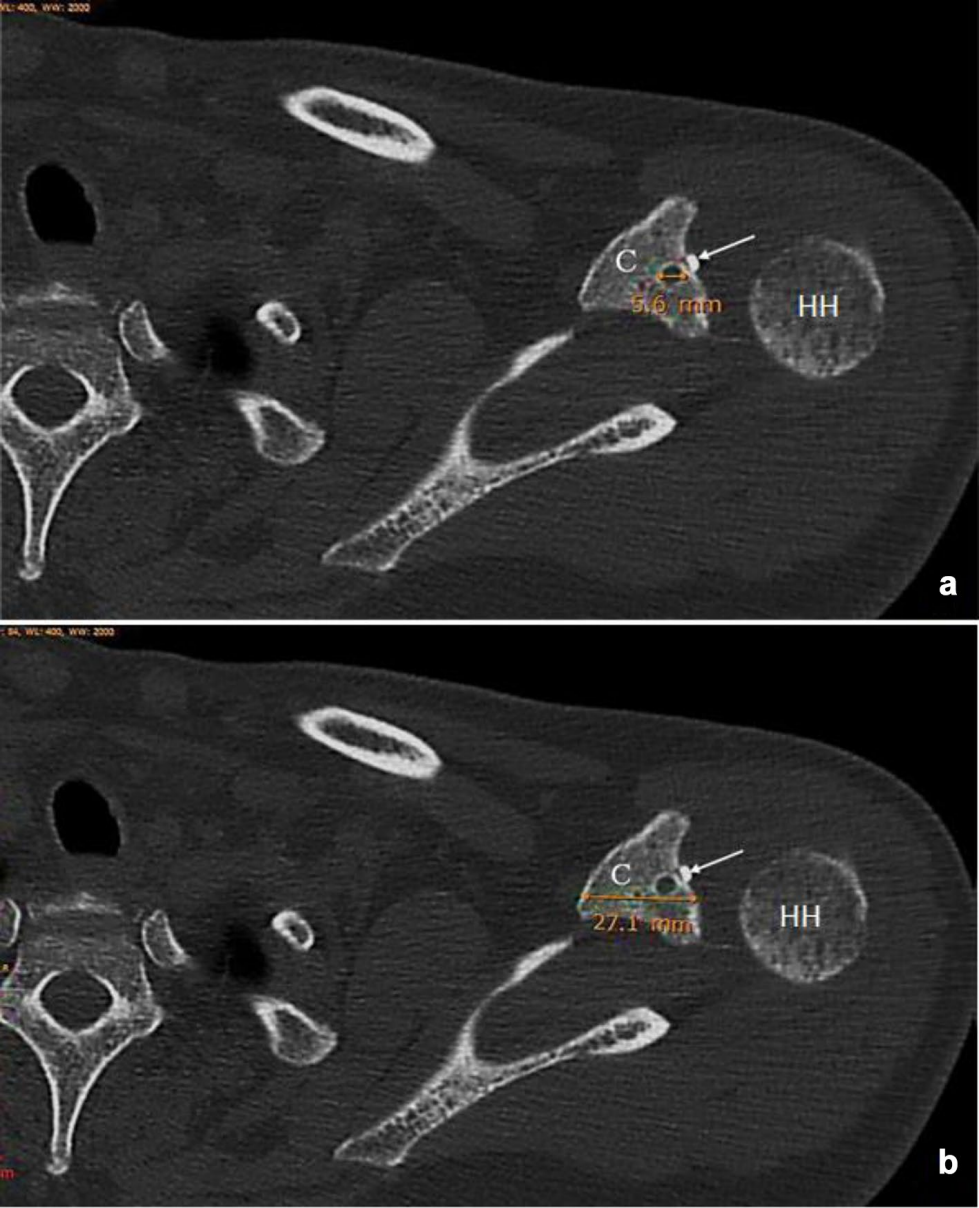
One-year postoperative CT scan axial cuts measuring a coracoid tunnel diameter at its widest width of 5.6 mm (orange line) and b coracoid horizontal diameter at its widest width of 27.1 mm (orange line). The ratio between both diameters was then calculated; Key: *C* coracoid, *HH* humeral head, white arrow for the endoutton
